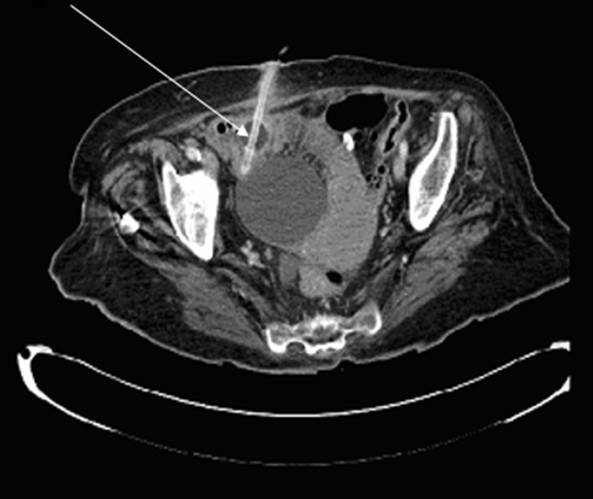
Axial computed tomography image demonstrating suprapubic catheter in situ of small bowel and balloon inflated causing partial small bowel obstruction (white arrow). The bladder appears uninterrupted.
